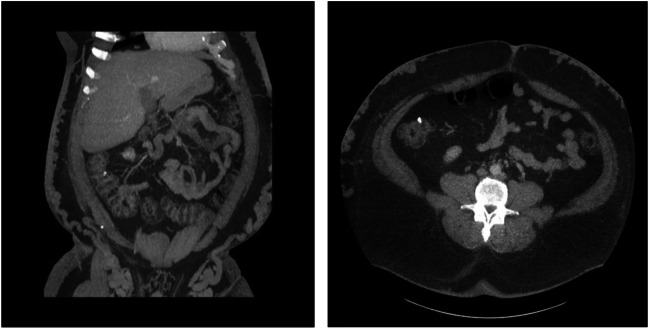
CT demonstrating venous collaterals arising from the common iliac veins on both sides and running along the flanks to the azygos and just below the skin in the subcutaneous plane
